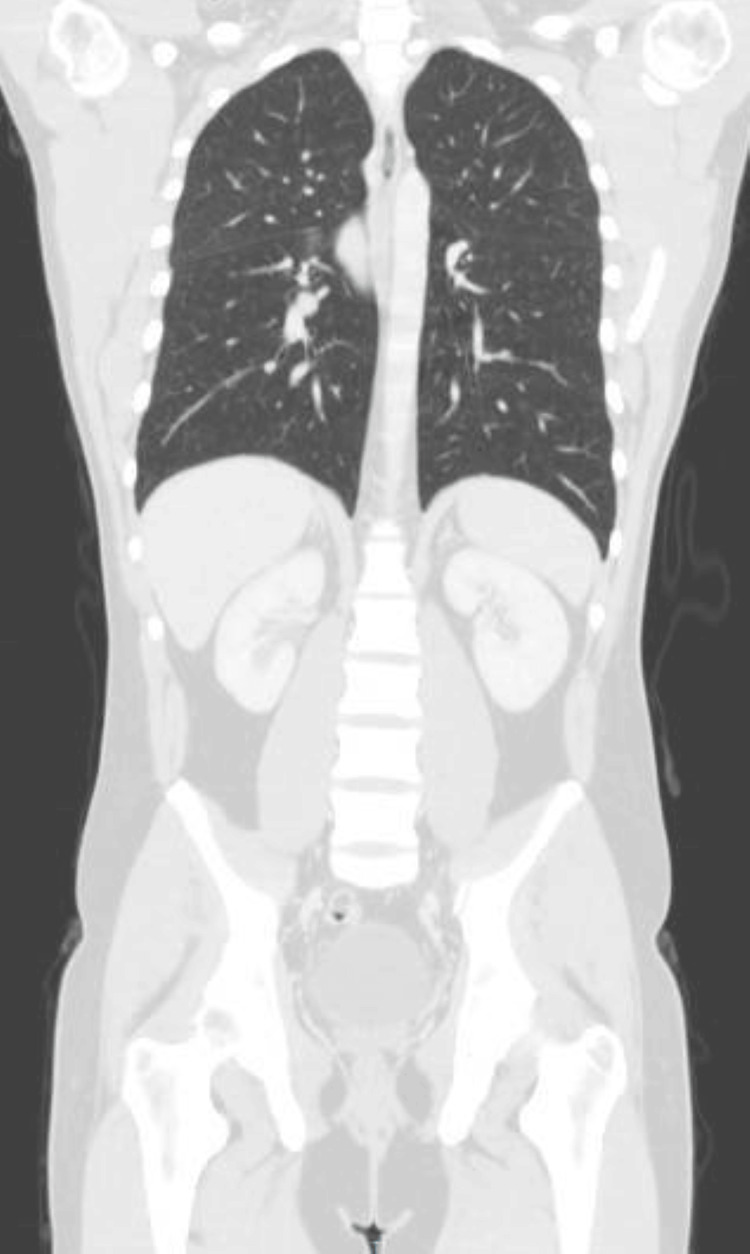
CT scan showing perihilar and mediastinal lymphadenopathy
